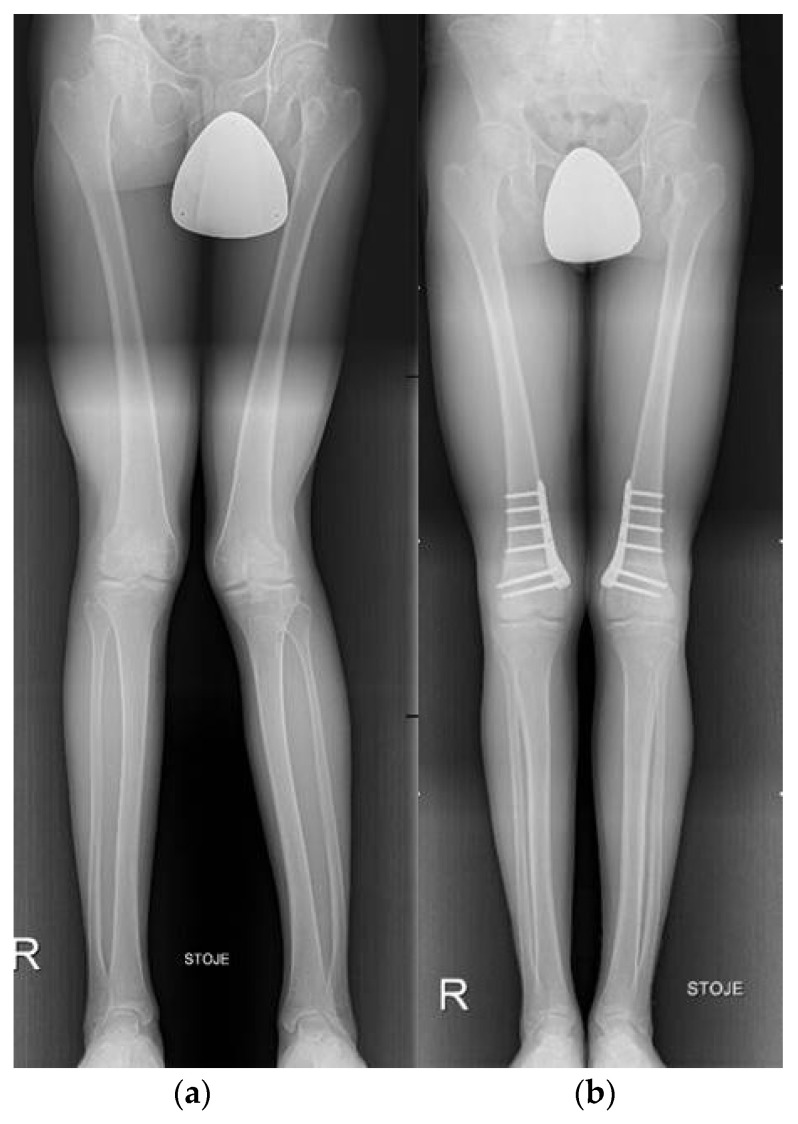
Bilateral distal femoral osteotomy (DFO). (a) preoperative X-ray of bilateral limb torsional deformity; (b) postoperative X-ray after bilateral DFO.
